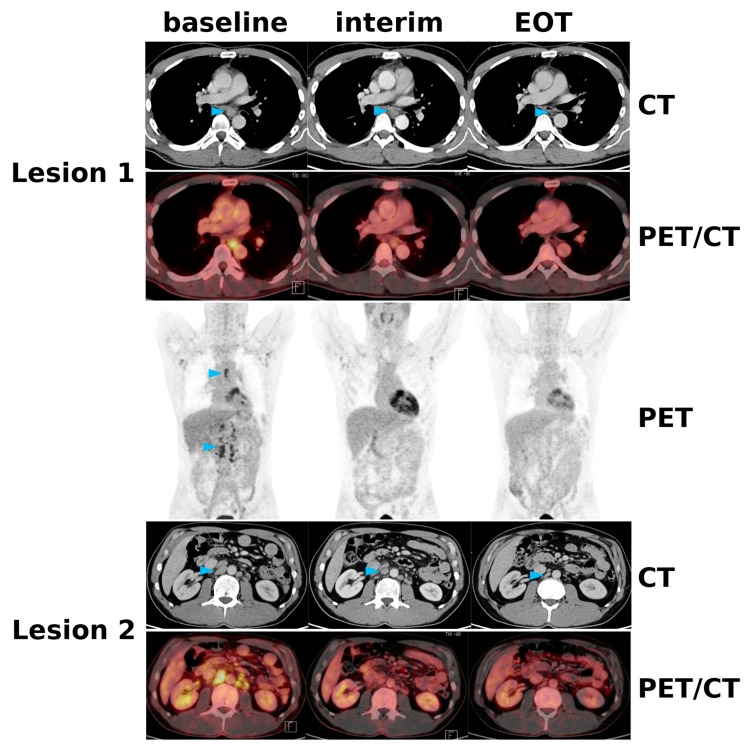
A 43-year-old patient with FL involving mediastinal and retroperitoneal (paraaortic) lymph nodes (blue arrowheads), as well as possible involvement of axillary and iliac lymph nodes. At interim restaging after three cycles of R-BENDA, the [^18^F]FDG uptake had decreased to Deauville ≤3 (i.e., not exceeding the liver uptake) in all nodal regions, which fulfills the Lugano criterion for CR. However, since the sum of the longest diameters (SLD) had only decreased by 24%, this represents MiR according to RECIL (Response Evaluation Criteria in Lymphoma) at interim restaging. At end-of-treatment (EOT), the SLD had finally decreased to 50%, consistent with CR according to both RECIL and Lugano.
